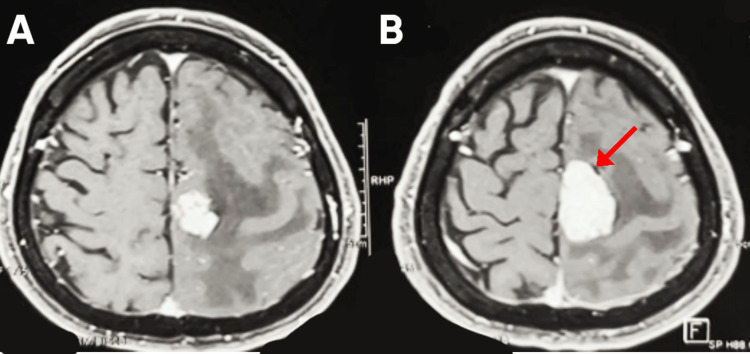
Preoperative axial post-contrast T1-weighted MRI of the brain (A) Axial image showing a left parasagittal dural-based lesion with homogeneous enhancement and associated mass effect. (B) Axial image demonstrating the same lesion (red arrow) compressing the adjacent motor cortex, consistent with a parasagittal meningioma.
